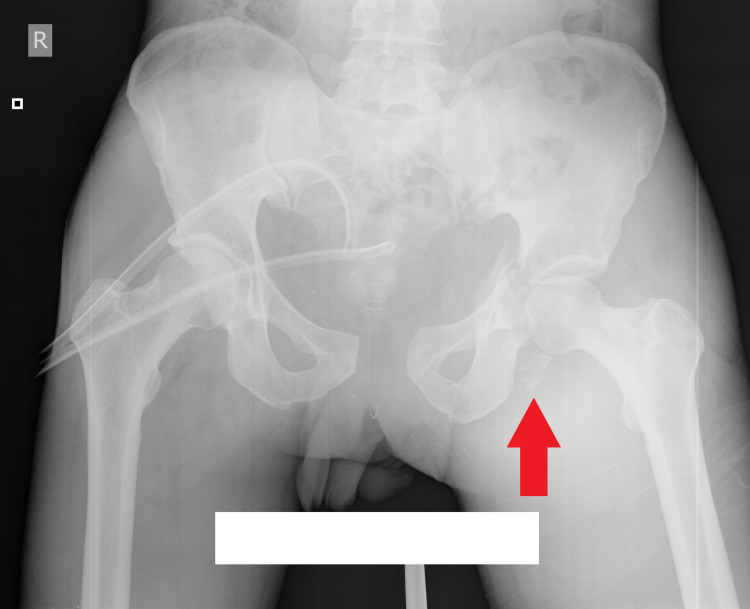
X-ray after closed reduction of left hip joint. The arrow shows markedly displaced posterior wall of acetabulum. Left side pelvic ring fracture (type B1.1 according to the Orthopaedic Trauma Association {OTA} classification), transverse undisplaced fracture of the right acetabulum, left acetabular transverse + posterior wall (according to Judet and Letournel classification) are present.
